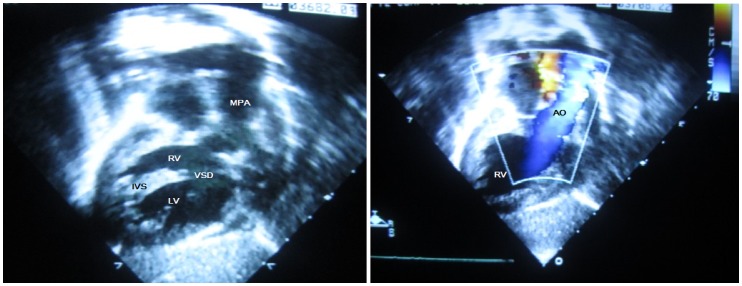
The echocardiography images of this case. MPA: main pulmonary artery, RV: right ventricular, VSD: ventricular septal defect, IVS: inter ventricular septum, LV: left ventricular, AO: aortic artery.
